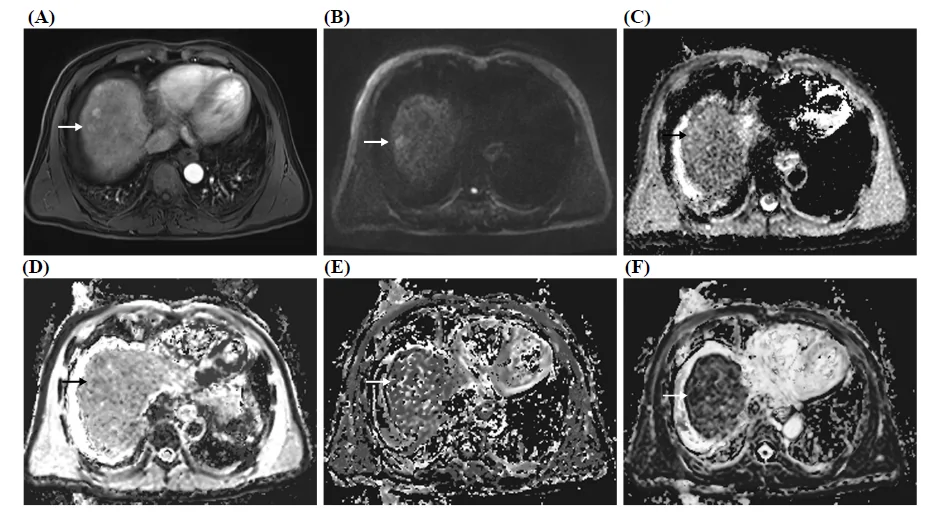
MR images in a 62-year-old man with recurrent HCC. Axial (A) enhanced fat-suppressed T1-weighted MR image shows a residual enhancing lesion with a nodular pattern (arrow) in the liver. Axial (B) DWI map shows a high homogeneous signal intensity. Axial (C) ADC map shows a low homogeneous signal intensity. Axial (D) * D* map shows a low homogeneous signal intensity. Axial (E) * D map shows a heterogeneous isointense signal. The axial (F) f map shows a low, homogeneous signal intensity. The calculated mean values of ADC, *D, D, and *f* for the recurrent HCC were 0.68×10^-3^ mm^2^/s, 0.88×10^-3^ mm^2^/s, 46×10^-3^ mm^2^/s, and 22%, respectively.
